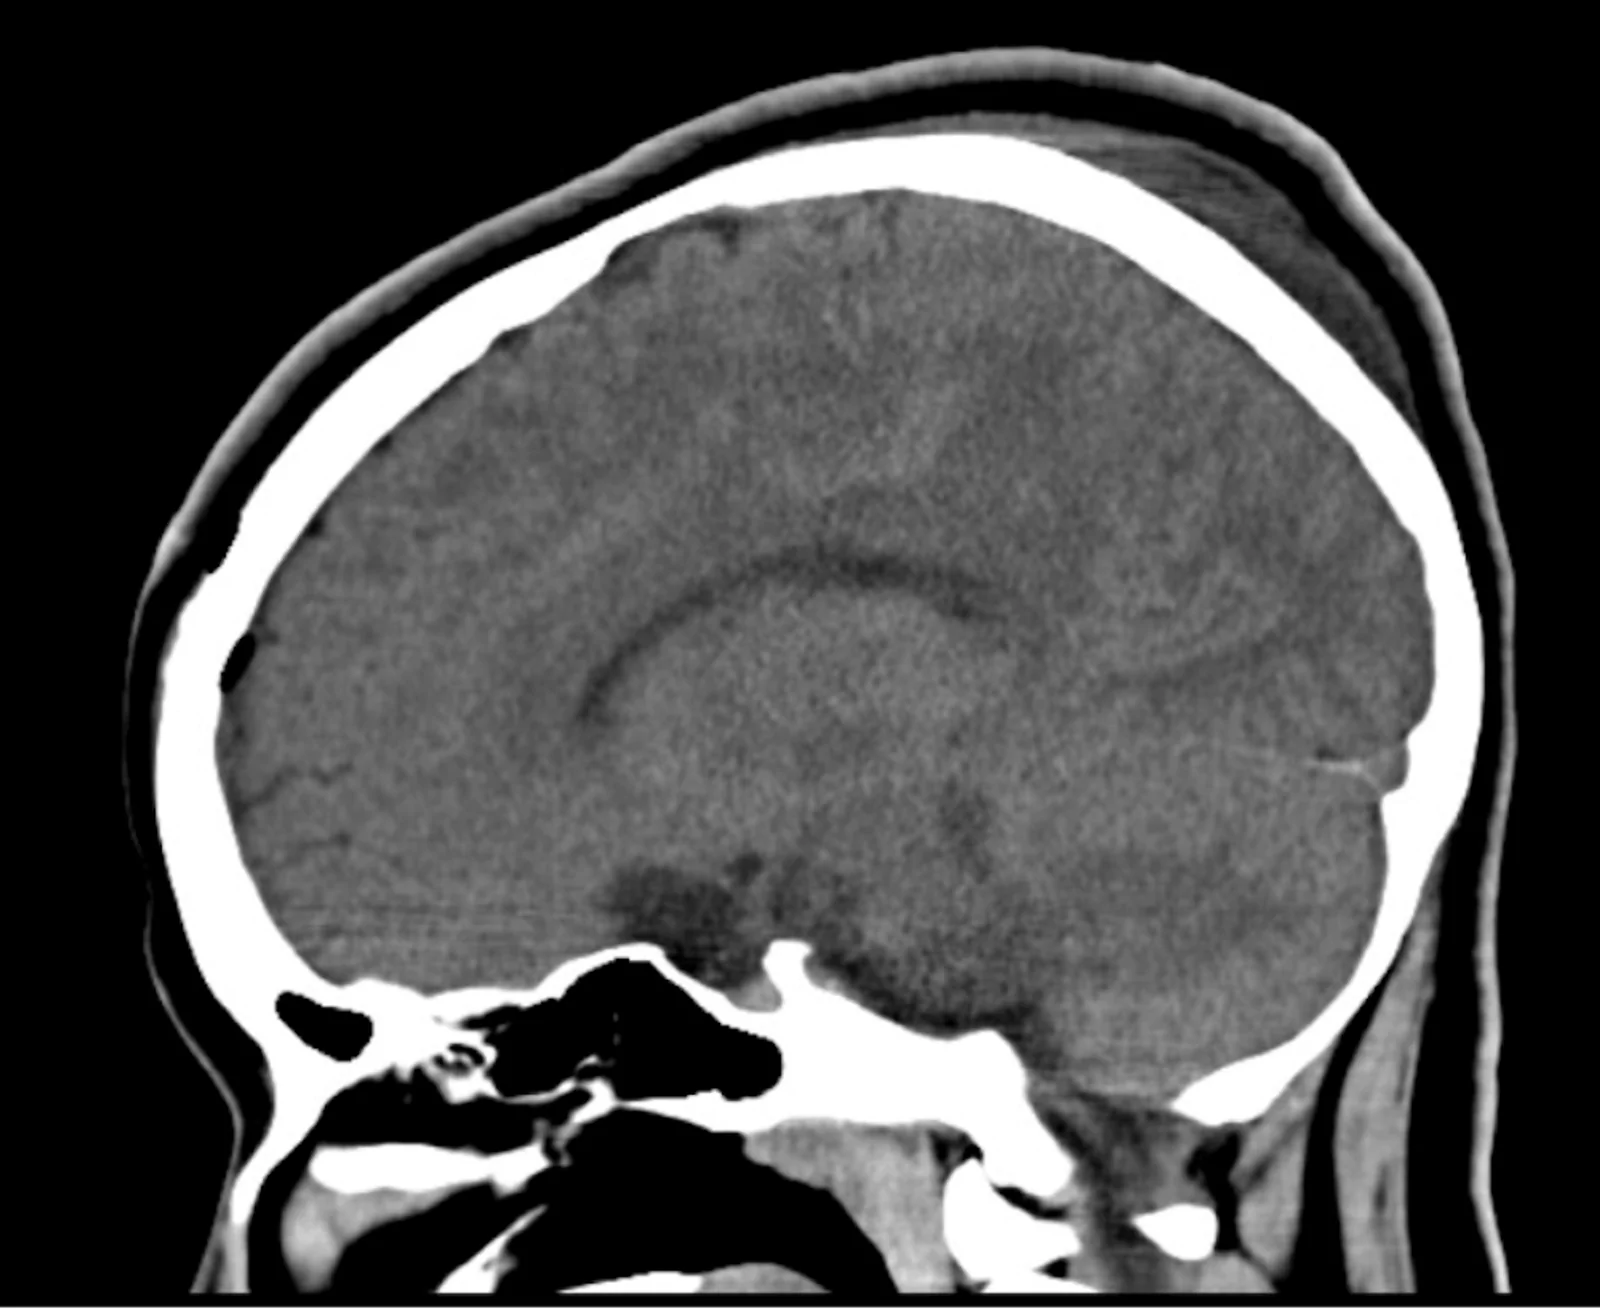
Postoperative sagittal CT of the head without contrast Imaging obtained after right frontotemporal craniotomy and resection of the lesion. CT: computed tomography
